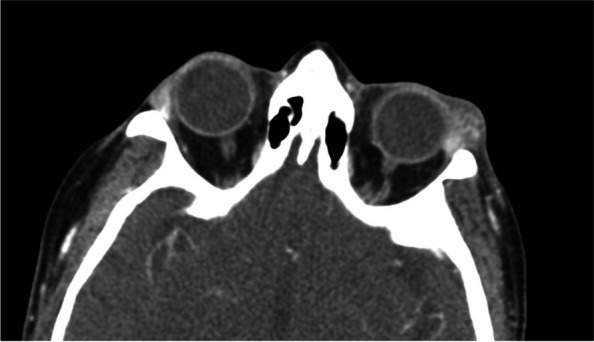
Axial CT scan of the orbits depicting left sided lacrimal gland enlargement
